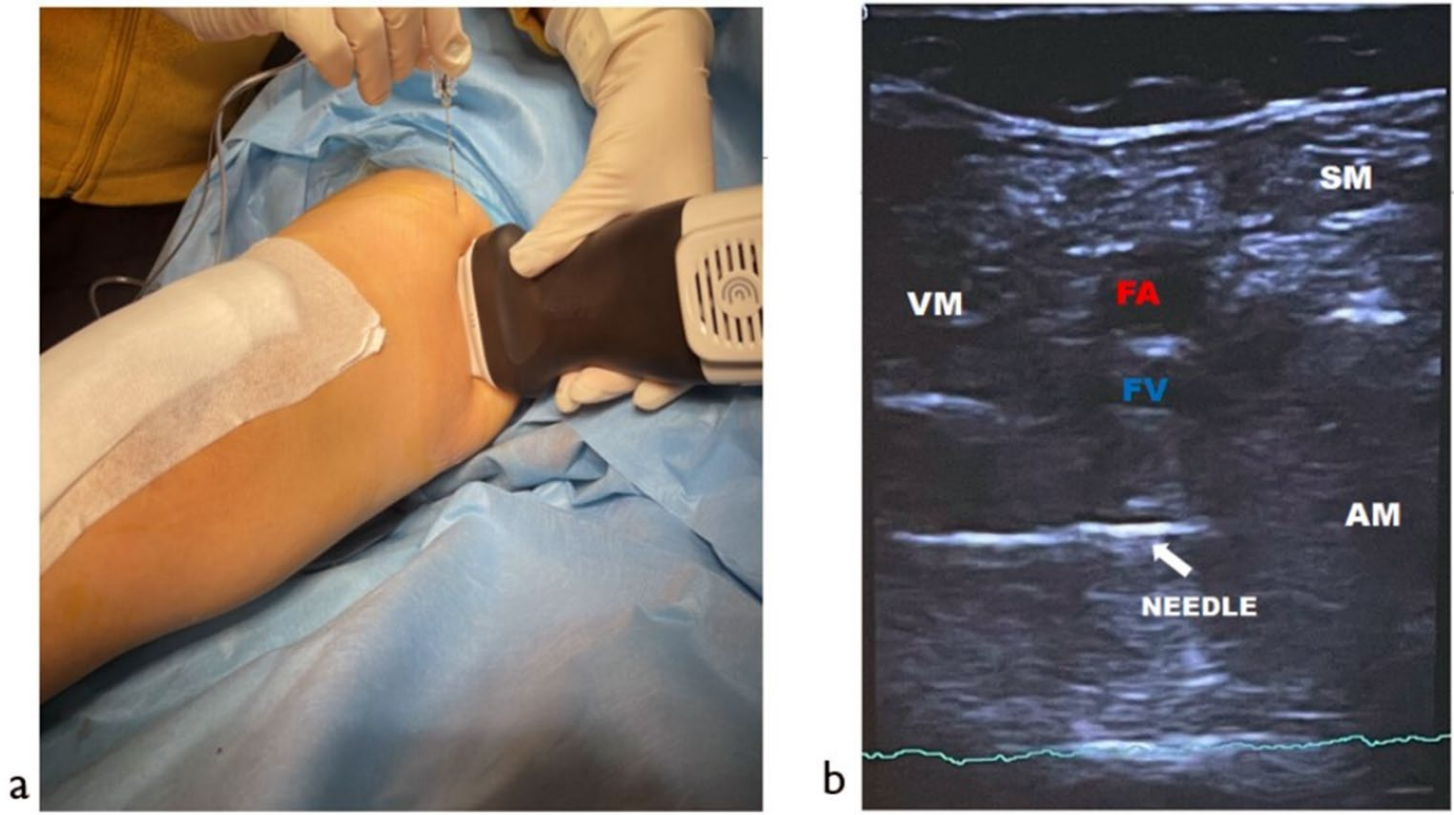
a) Postoperative ultrasonographic visualization adductor blockage with sterile condition. b) FA - Femoral Artery, FV - Femoral Vein, VM - Vastus Medialis Muscle, S - Sartorius Muscle, AM - Adductor Magnus Muscle
